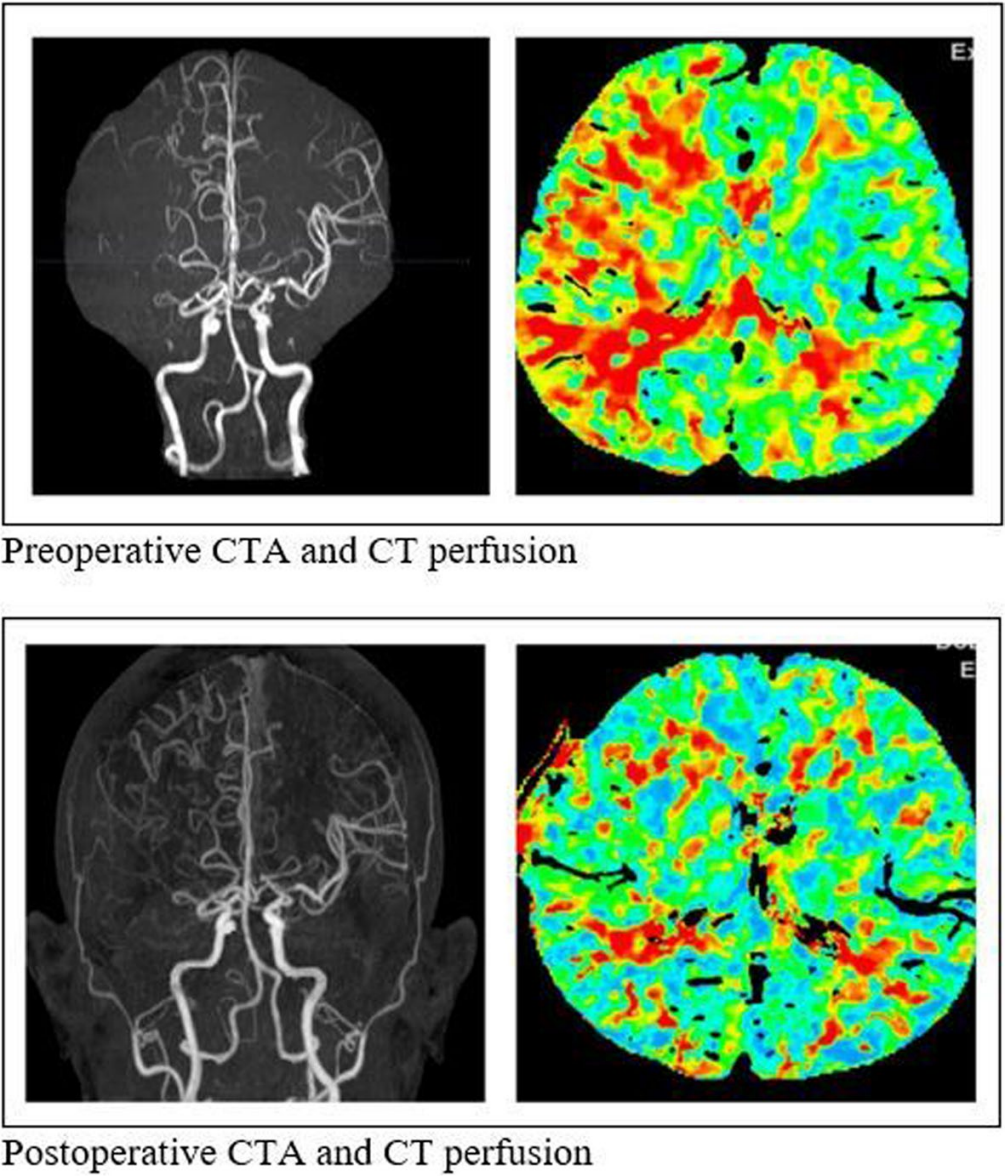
Pre-Postoperative CTA and CT perfusion
